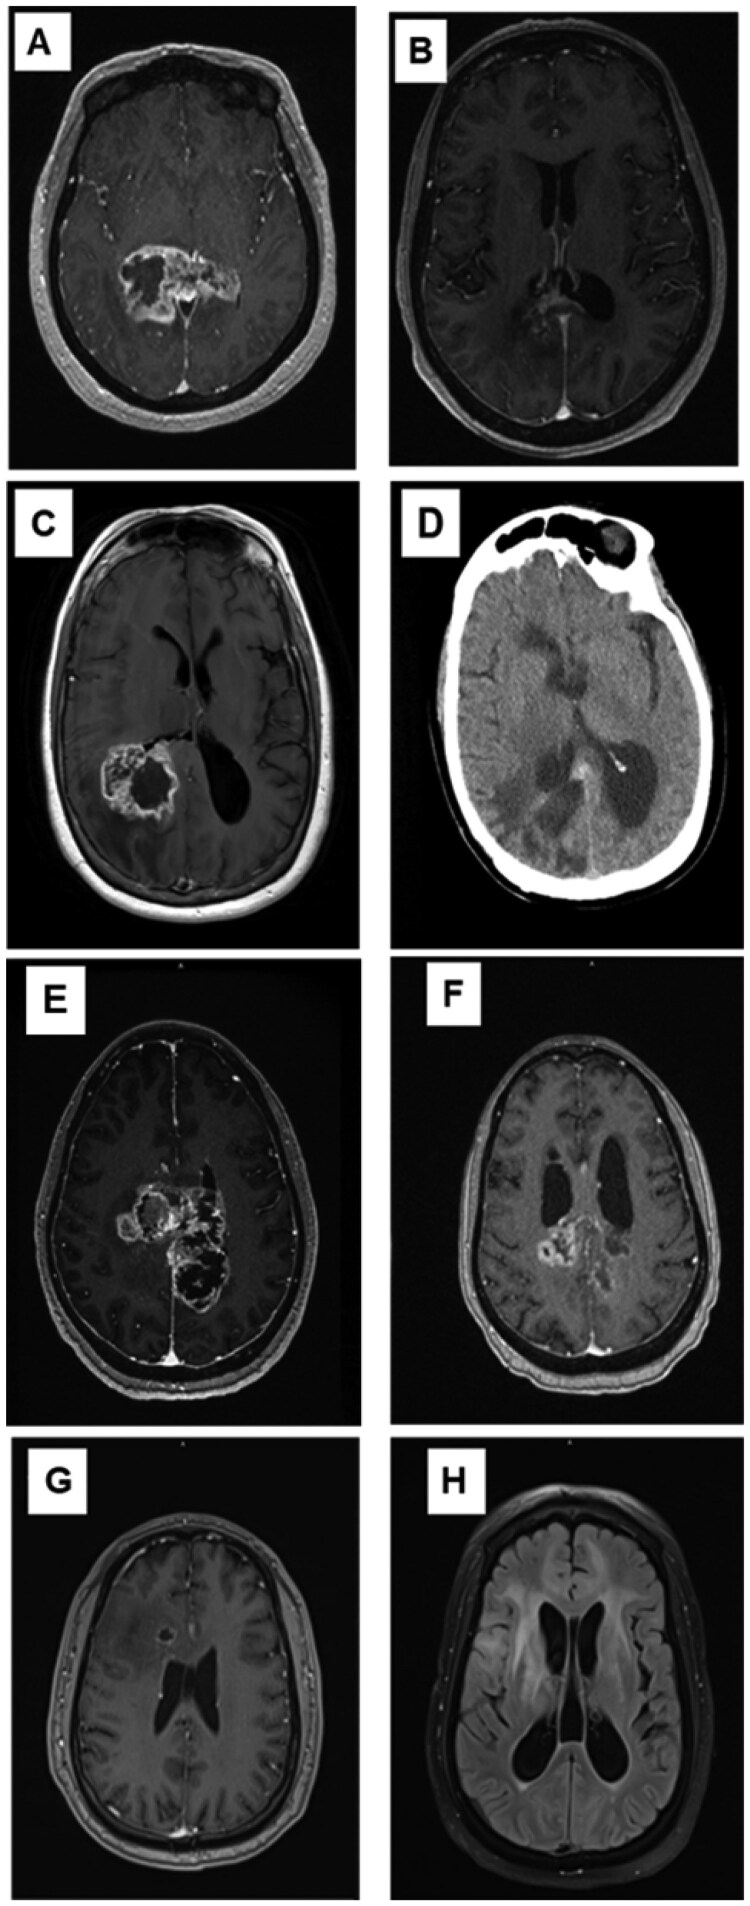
MRI of patients 1, 3, 4, and 5 at baseline and after tepotinib initiation. (A) Patient 1 scan at baseline (December 11, 2023). (B) Patient 1 scan at the latest follow-up post-tepotinib initiation (June 27, 2025). (C) Patient 3 scan at baseline (August 31, 2023). (D) Patient 3 scan after 3.7 months of tepotinib (June 2, 2024). (E) Patient 4 scan at baseline (January 23, 2024). (F) Patient 4 scan 3 weeks after tepotinib initiation. (G) Patient 5 scan at baseline (February 26, 2023). (H) Patient 5 post-contrast and T2 FLAIR images after 0.7 months of tepotinib treatment (September 25, 2023). MRI, magnetic resonance image.
